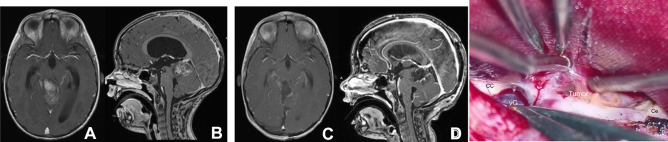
A 5-year-old girl with exophytic tectal JPA. Post-contrast MR images, before (A axial, B sagittal) and after (C axial, D sagittal) resection show a heterogeneously enhancing tumor extending to the quadrigeminal cistern. Surgical photo showing a view of an exophytic tumor through the tentorial opening (cc: corpus callosum, vG: vein of Gallen, Ce: cerebellar vermis)
